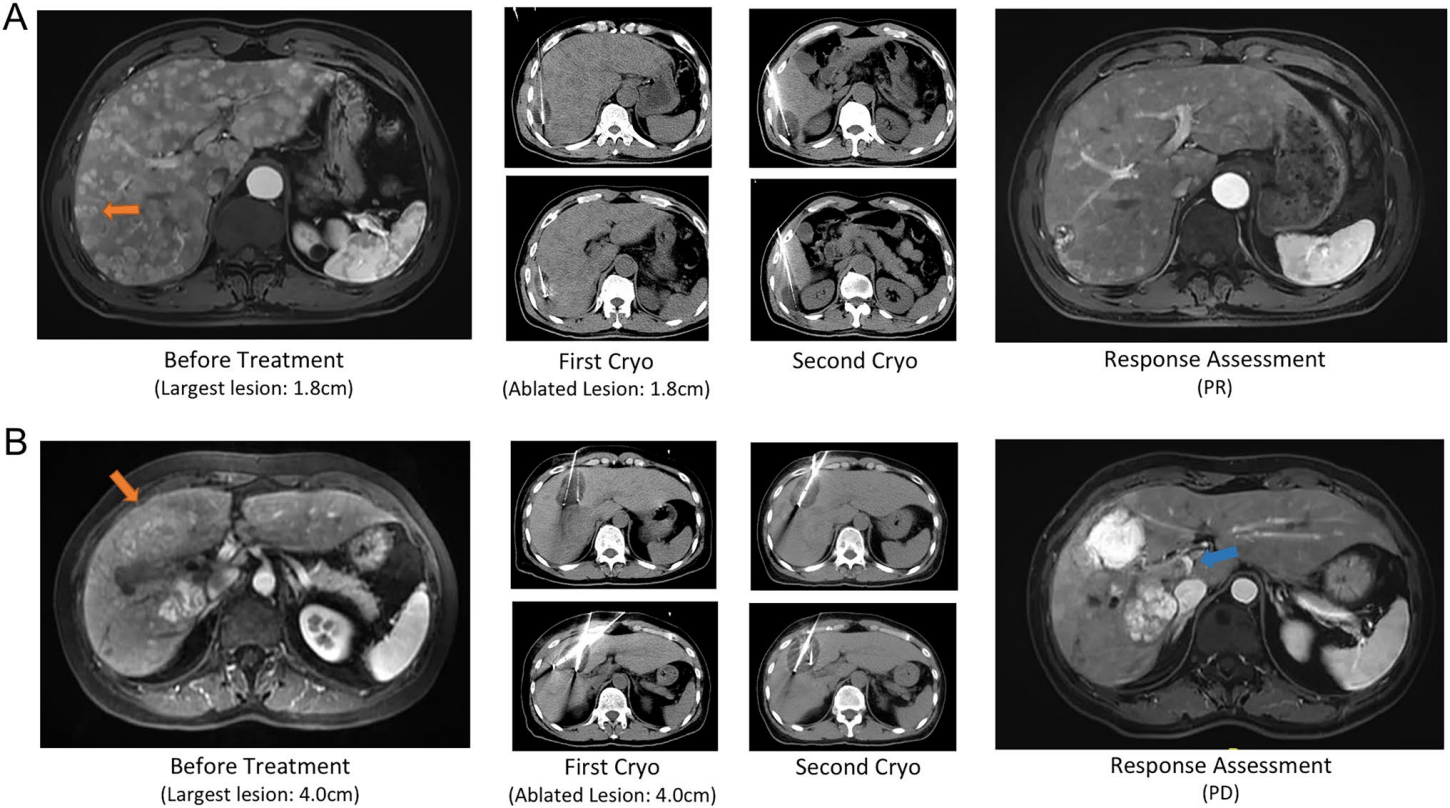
Typical cases of melanoma patients with different sizes of intrahepatic tumors receiving combined cryoablation and PD-1 blockade therapy. A An adult patient with cutaneous melanoma had progression in liver metastasis after two cycles of pembrolizumab therapy. Contrast-enhanced T1-weighted imaging (T1WI) showed that a major PR was achieved after two cycles of combination treatment; CR was achieved after two more cycles of pembrolizumab infusion (arrow, target lesion for the 1st cryoablation). B An adult patient was diagnosed of multiple liver and bone metastases after resection of uveal melanoma. She received two cycles of combination treatment while contrast-enhanced T1WI showed unablated intrahepatic tumors and protal vein tumor thrombus (PVTT) progressed continuously (orange arrow, target lesion for cryoablation and blue arrow, PVTT)
